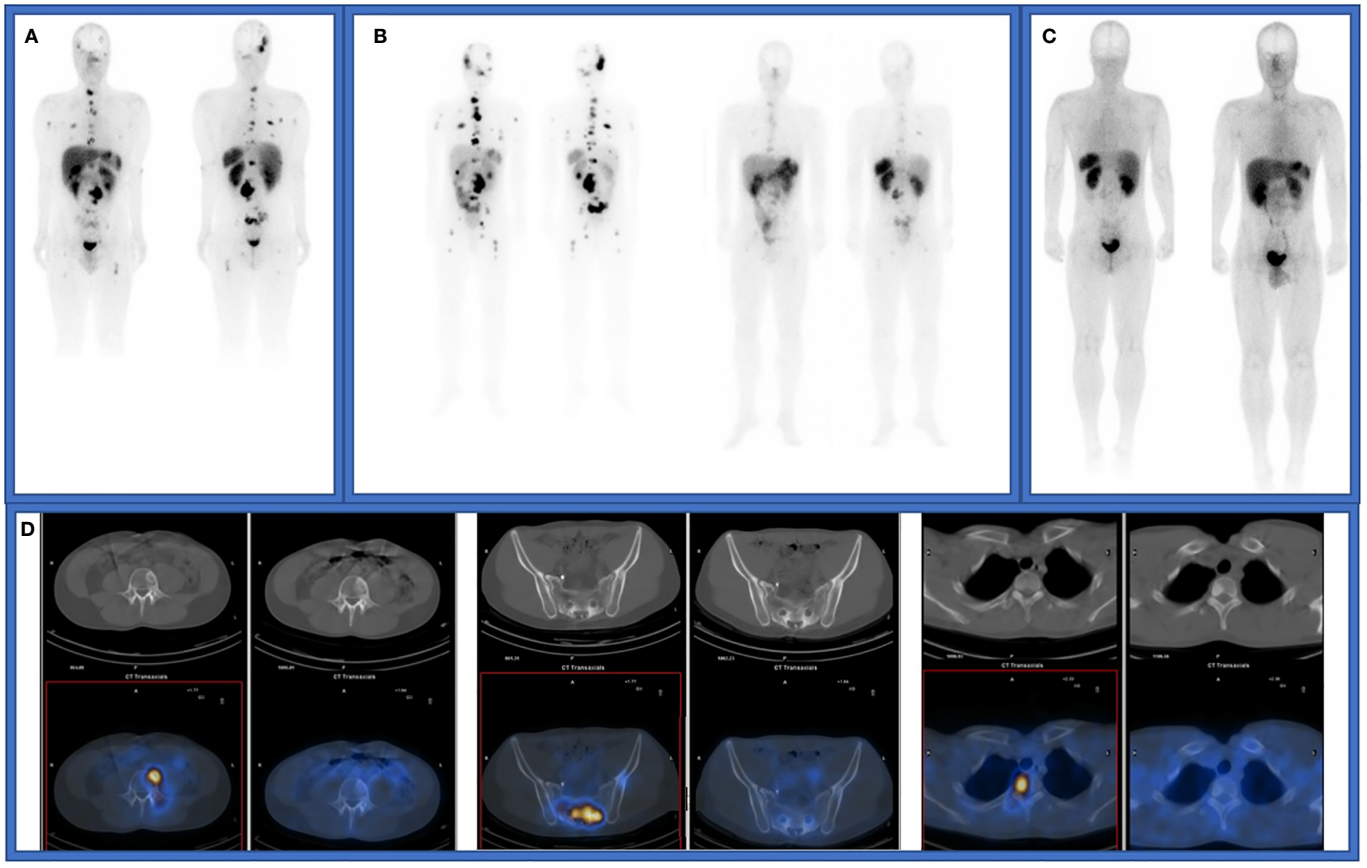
(A) Octreoscan imaging in December 2015, before de first treatment with 177Lu-DOTATATE. (B) SPECT-CT studies after first and fourth 177Lu-DOTATATE doses. (C) Octreoscan imaging in November 2016, after finishing first treatment with 177Lu-DOTATATE. (D) SPECT-CT studies in December 2015 and November 2016.
